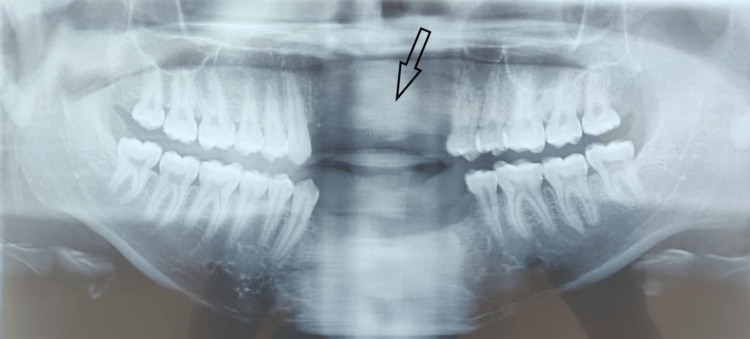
Preoperative radiograph after eight months of road traffic accident
